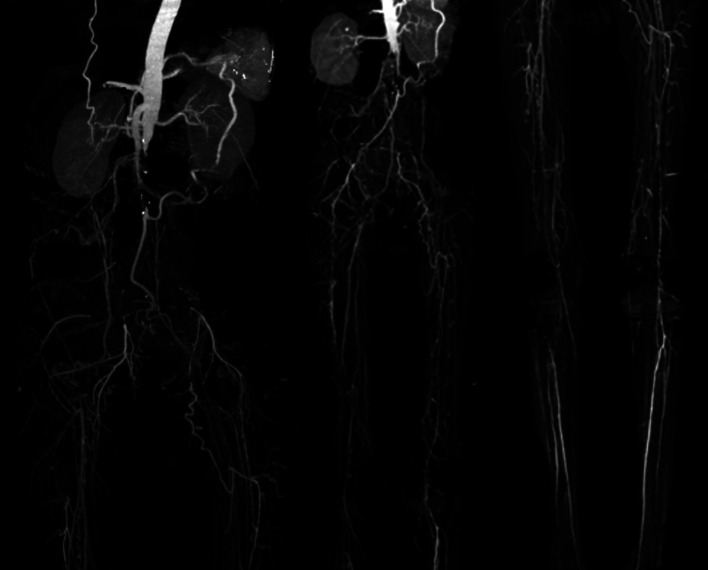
Lower extremity CT Angiography of 2
